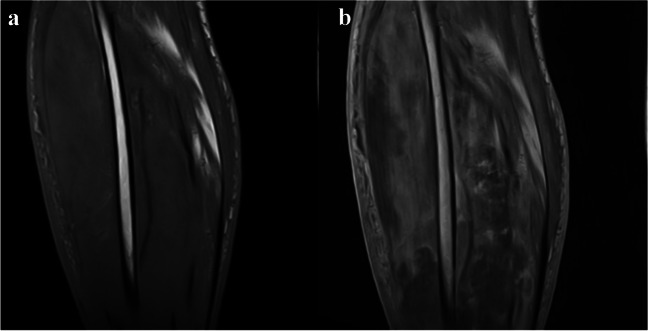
Pre-contrast (a) and post-contrast (b) T1-weighted MRI images, depicting band-like enhancement at the periphery of the muscles involved in the lower leg
